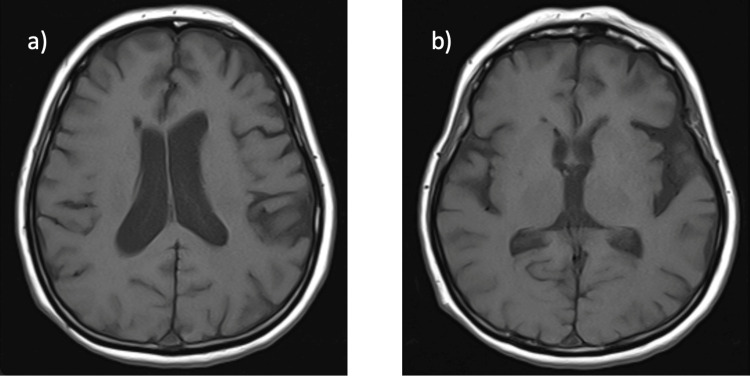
The axial T1 MRI showing cerebral atrophy, bilateral lateral ventricle dilatation (a), and third ventricle dilatation (b).
